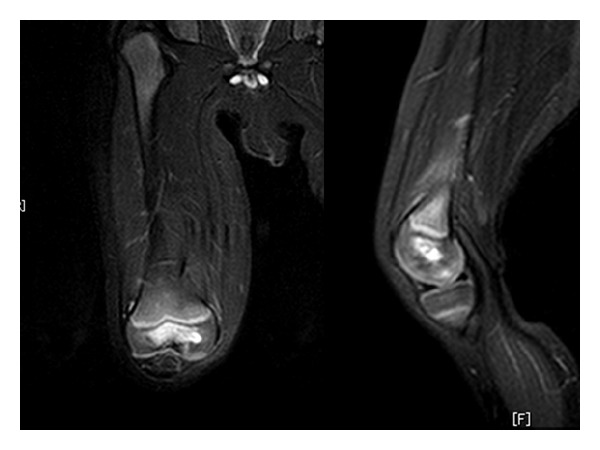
STIR sequences on MRI two weeks after treatment show that bone marrow abnormality and soft tissue edema of the femur have improved. Note the high intensity area in the epiphysis, especially in the medial epiphyseal nucleus.
